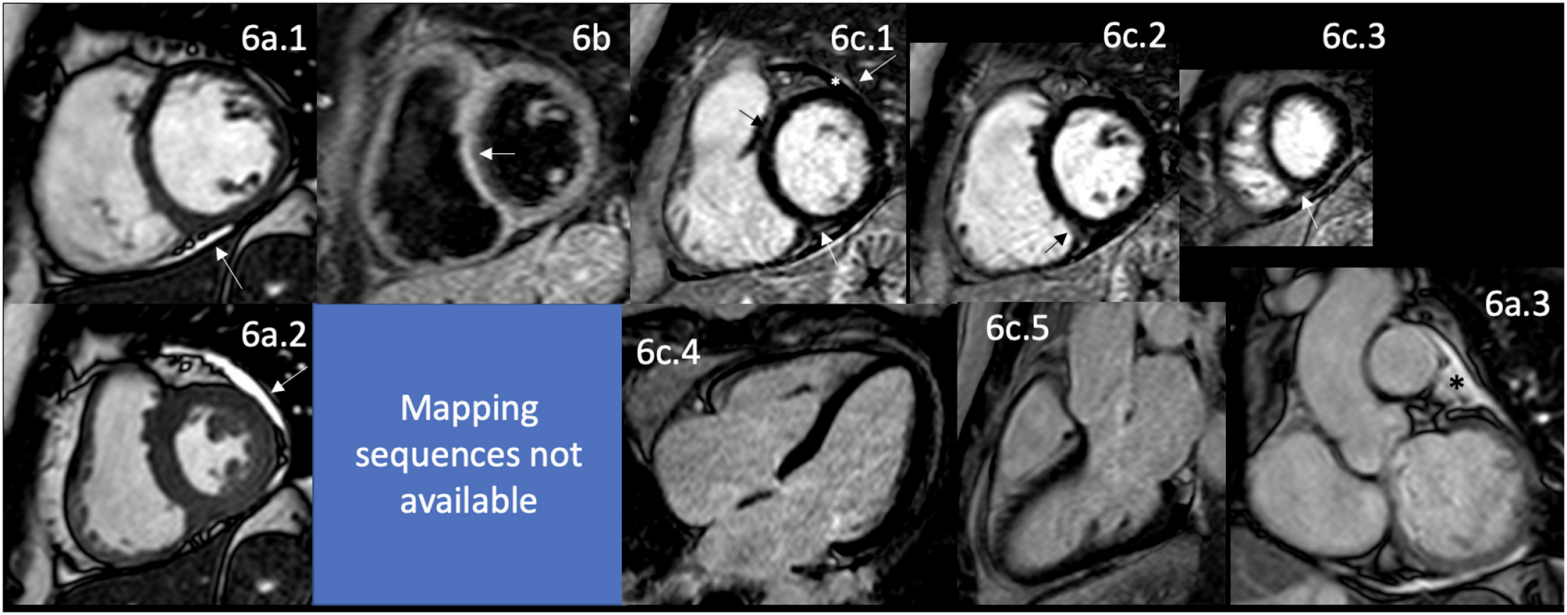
CMR findings in DS. A panel figure demonstrates varying findings from a comprehensive CMR study at a 1.5 Tesla scanner of DS. (a) SSFP sequence still cine images in short-axis view at the mid-ventricular level in end-diastole (a.1) and end-systole (a.2) show normal global and regional functions of the left ventricle, LVEF 63% and mildly reduce systolic global RV function and mild global hypokinesia RVEF 45%. (a.3) In a coronal view showing the mild pericardial effusion surrounding the heart (asterisks). (b) T2-W STIR sequence in short-axis view at a mid-ventricular level showing increased signal intensity in the septal segments (arrow) with a myocardial/skeletal muscle ratio of 2.3, suggesting mild myocardial edema. (c.1–5) LGE PSIR sequence. (c.1–3) Short axis views at basal (c.1), mid (c.2), and apical (c.3) levels, and long-axis views in four-chambers (c.4) and three-chambers (c.5). The arrows in these images show areas of focal fibrosis as midventricular LGE of the anteroseptal and inferior segments in (c.1) and in the subepicardium of the inferior segment in (c.2,3). (c.1) Shows mild pericardial effusion (asterisks) and trivial pericardial enhancement (arrow), suggesting minimal pericardial inflammation. CMR, cardiovascular magnetic resonance; DS, DRESS syndrome; SSFP, steady-state free precession; LVEF%, left ventricular ejection fraction; RVEF%, right ventricular ejection fraction; T2-W STIR, T2-weighted short-tau inversion recovery; LGE, late gadolinium enhancement; PSIR, phase-sensitive inversion recovery.
